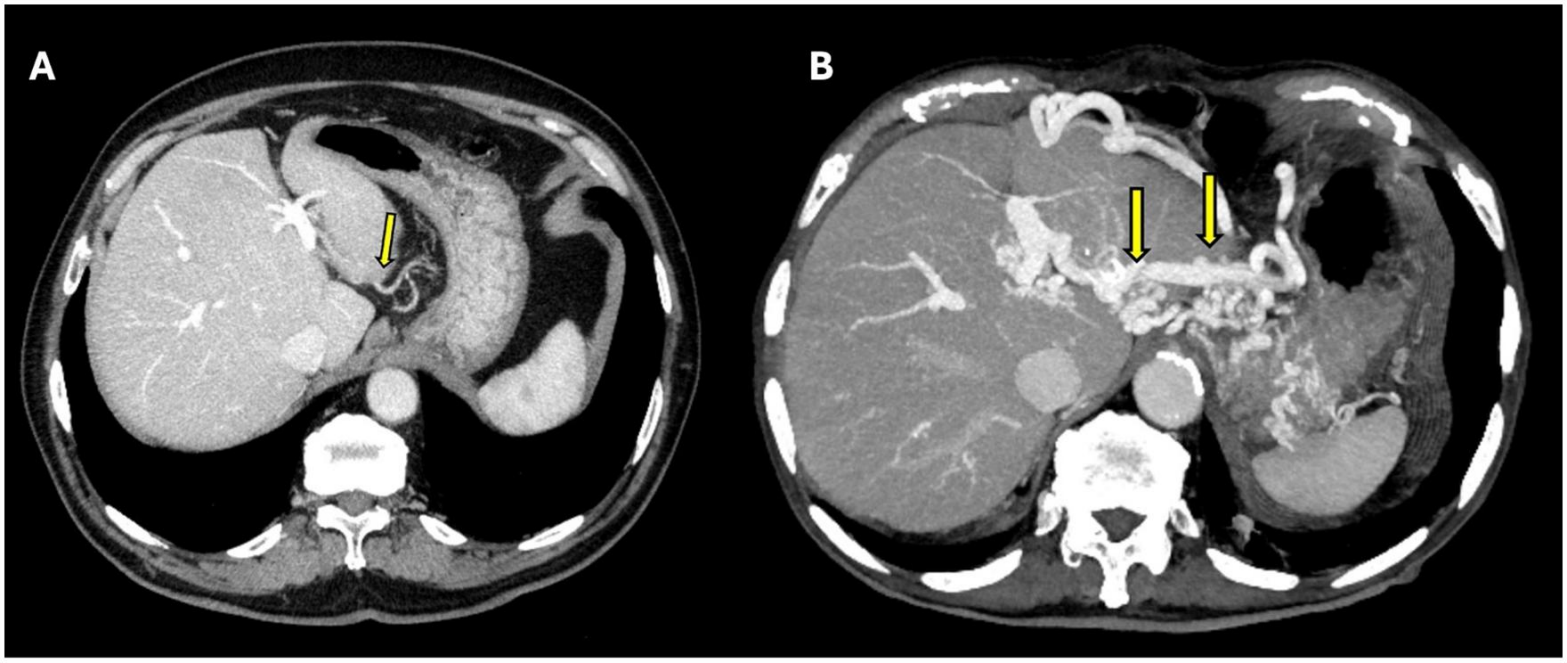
(A) Axial portal phase contrast-enhanced CT MIP image of a patient evaluated for pancreatic cancer demonstrates the aberrant left gastric vein draining into both segment 2 parenchyma and the left portal vein (arrow), consistent with Type 2 ALGV. (B) In the follow-up CT MIP image 1 year later, a collateral vessel secondary to pancreatic tumour invasion into the portal vein is observed. The development of collateral vessels makes Type 2 ALGV more apparent (arrows).
